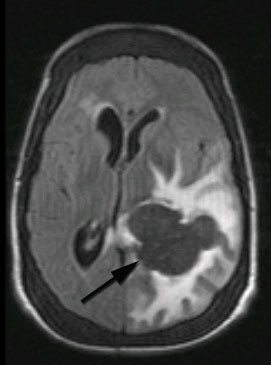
Axial T2-weighted MRI image showing a signal void brain lesion in the left trigone area. The surrounding vasogenic edema is well demonstrated.
